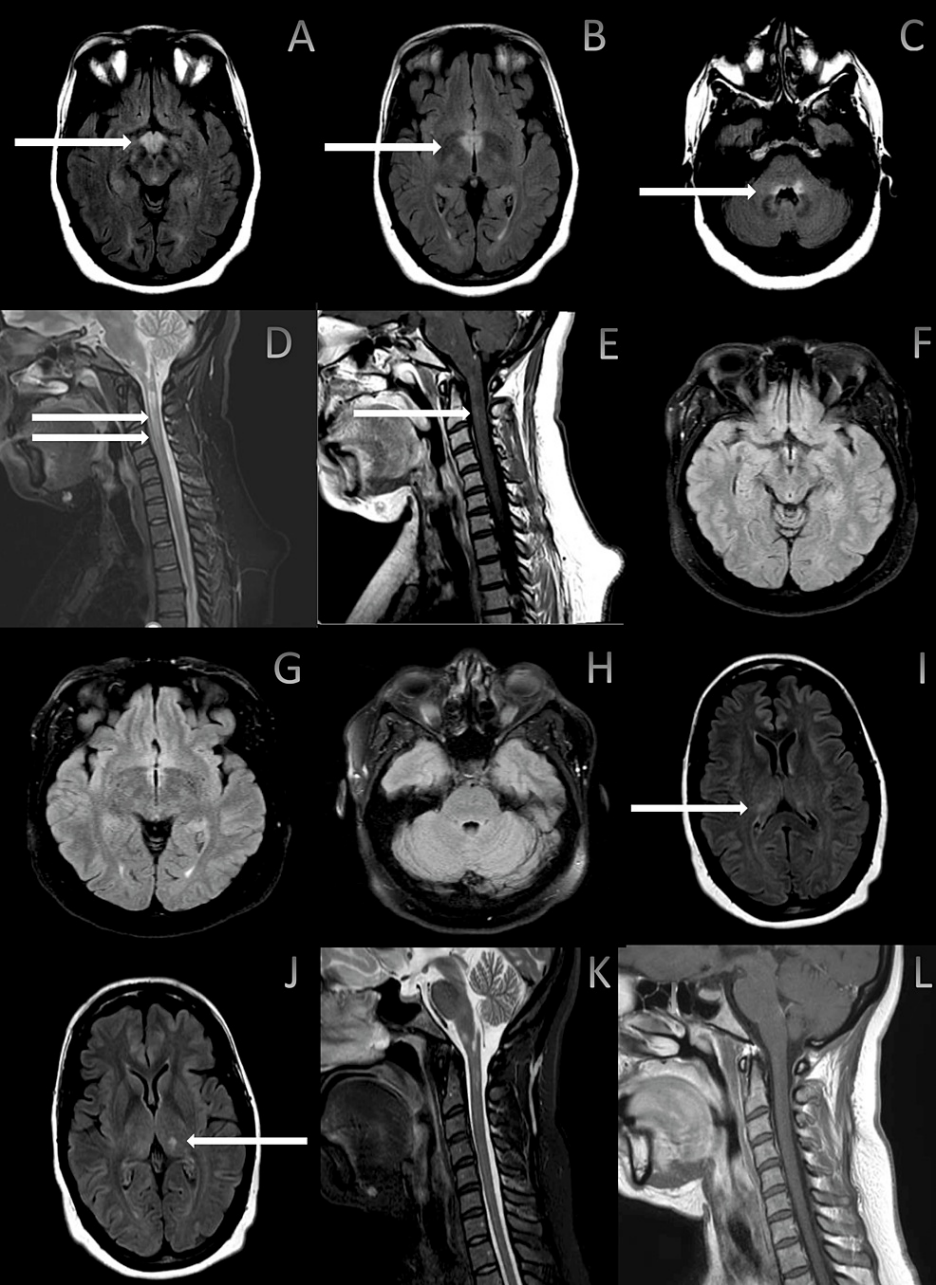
Magnetic resonance imaging (MRI) of the brain and cervical spine MRI of the brain fluid-attenuated inversion recovery axially showing T2 hyperintensities in the bilateral hypothalamic regions (A, B) and pontomedullary junction (C), with interval resolution imaging after a year (F-H). MRI of the cervical spine showing T2 signal changes from cervicomedullary junction to C3 level (D) with faint gadolinium enhancement (E). Repeat MRI of the brain, interval of two years, showing new T2 hyperintensities in the bilateral thalami (I, J). Repeat MRI of the cervical spine, interval of 18 months, showing improved T2 hyperintensities within cervical medullary junction (K), without enhancement (L)
